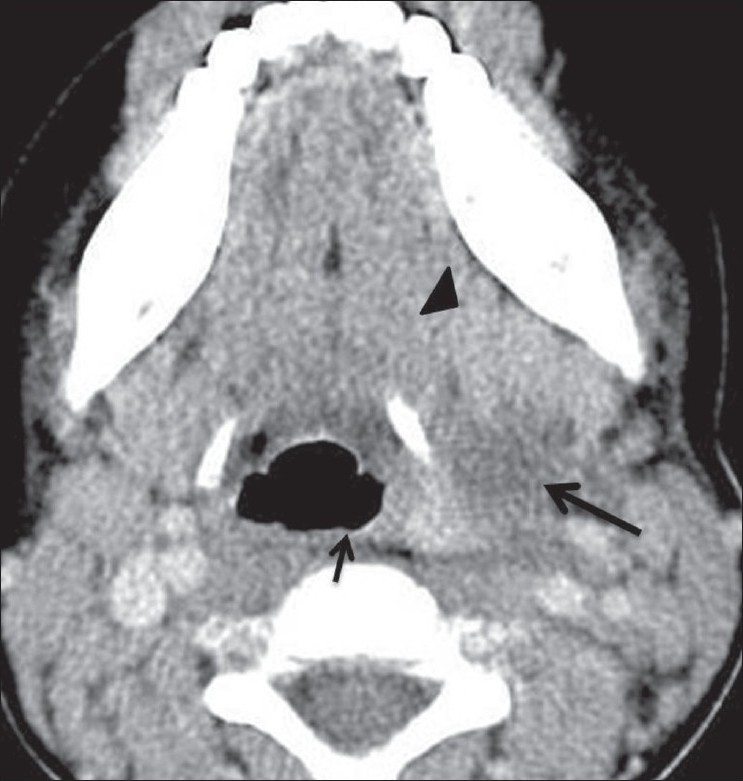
Axial CT scan of the neck shows the cranial (proximal) end of the lesion (arrow) and its relation to the left submandibular gland (arrowhead) and pyriform sinus (short arrow)
